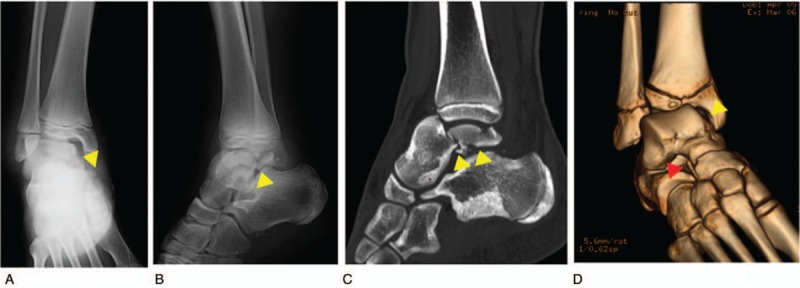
Preoperative radiographs and computed tomography (CT) (yellow arrow head: talus body fracture; red arrow head: avulsion fracture of navicular bone). (A) Anterior-posterior view. (B) Lateral view. (C) Sagittal image of CT. (D) 3-Dimensional reconstruction of CT.
